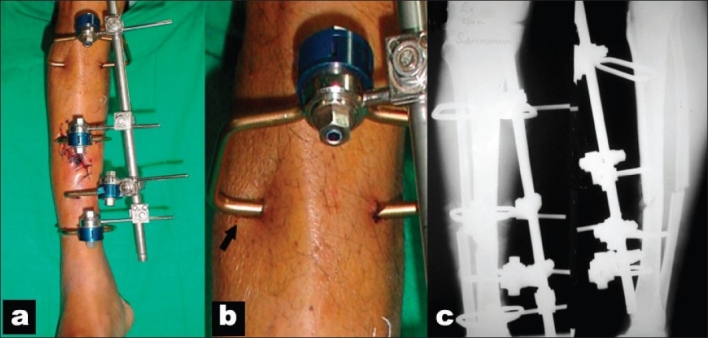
Pictures of pinless fixator used for stabilization of open fracture tibia. (a) An external fixator is in situ for an open fracture of tibia. (b) A close view of the pin site, with limb swelling almost subsided and the pressure on skin around the clamp relieved. (c) Postoperative radiograph shows well aligned fracture stabilized with four-clamp pinless fixator.
